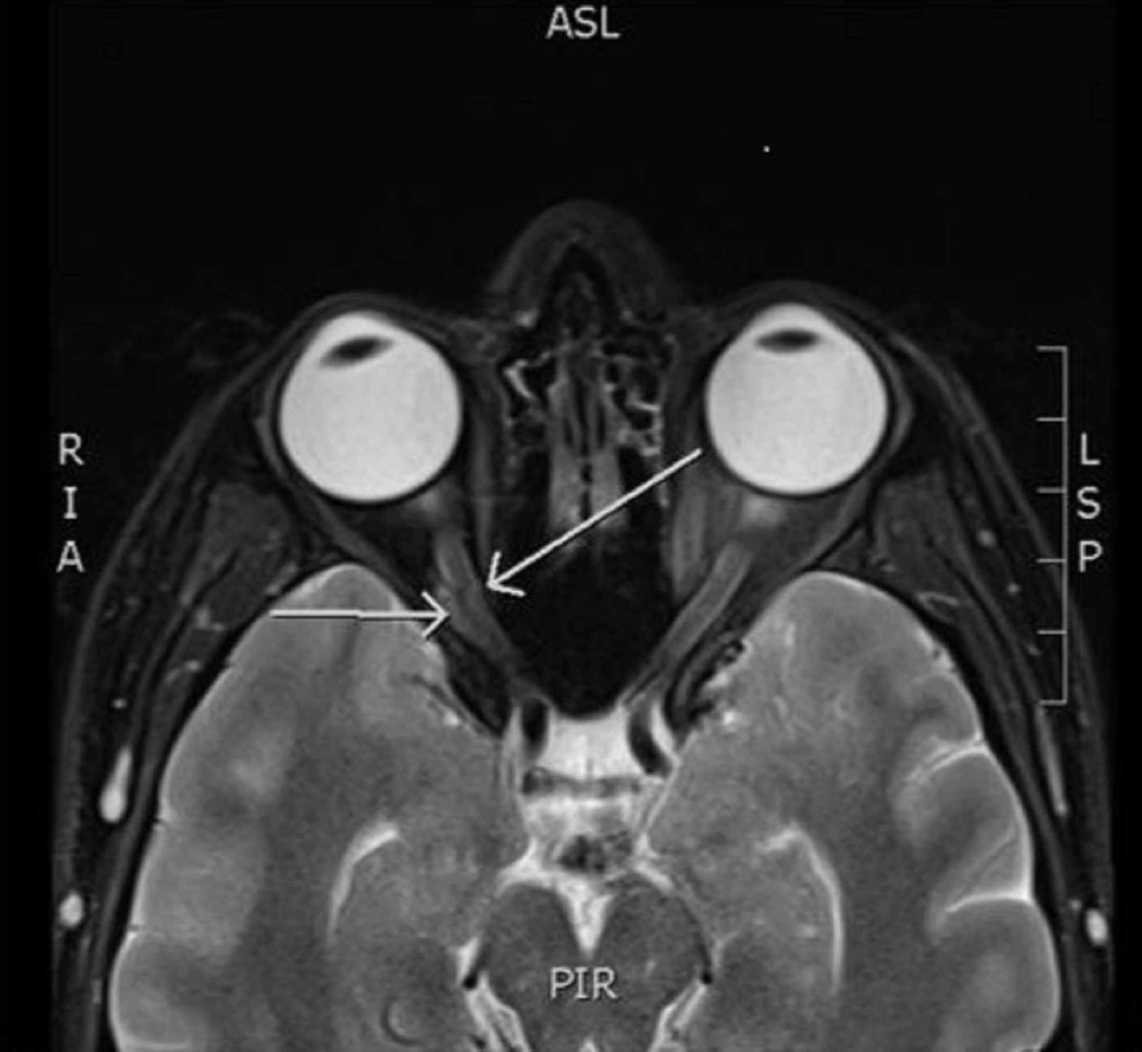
A transverse slice of a T2-weighted magnetic resonance image of the brain and orbits demonstrating right optic nerve sheath edema (white arrows).
